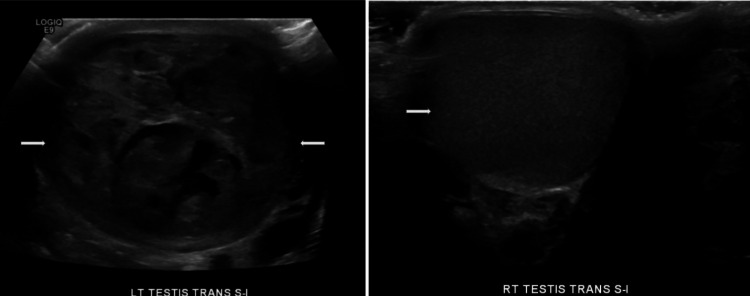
Ultrasound of the scrotum showing heterogeneous solid and cystic appearing left testicle (left) and normal right testicle (right)
